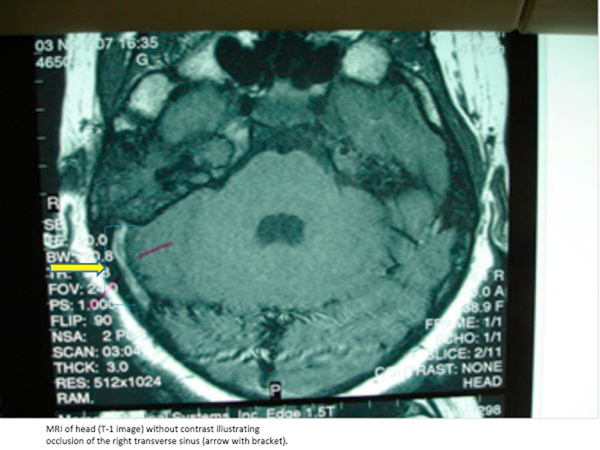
Magnetic resonance imaging of the head: Cord sign of the right transverse sinus (yellow arrow with bracket).
